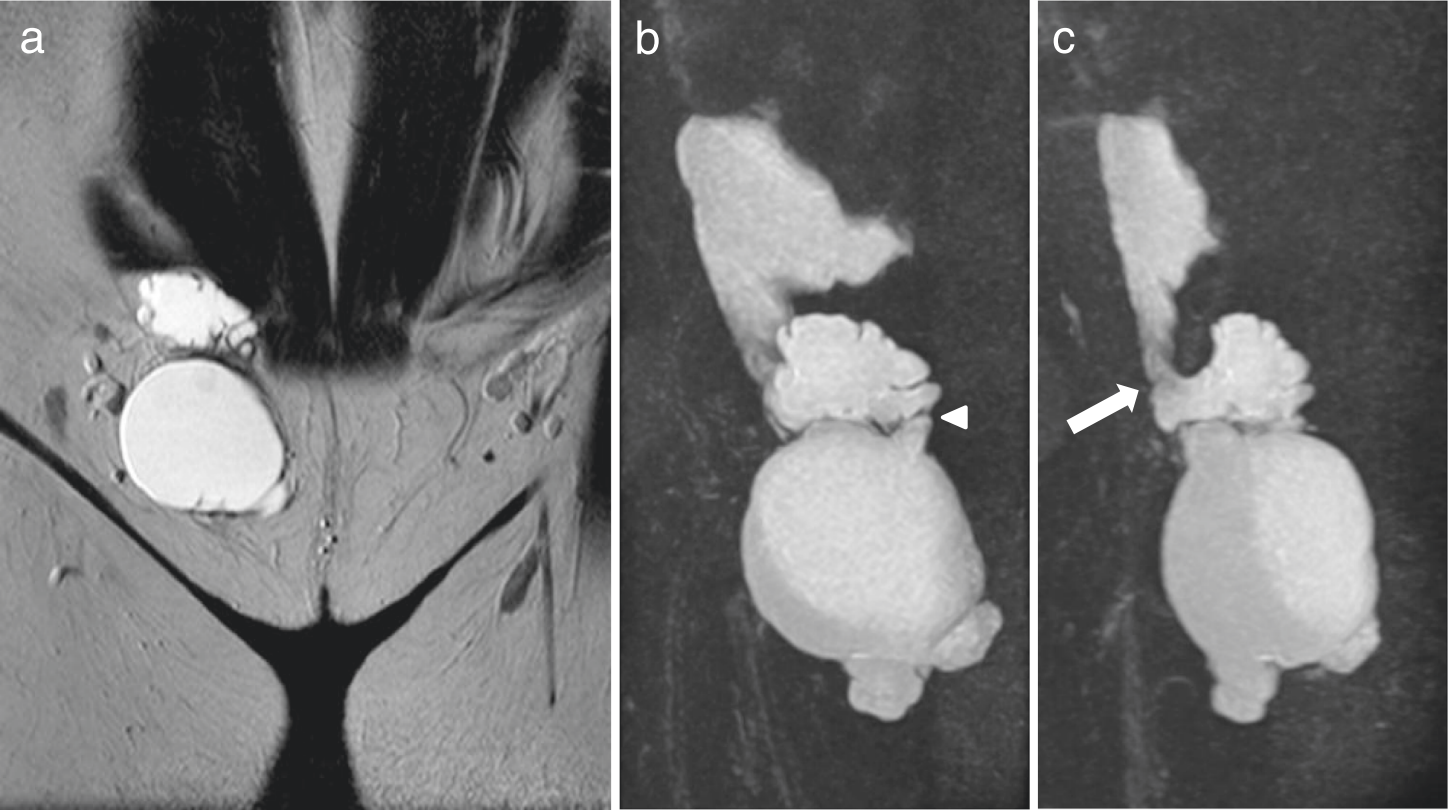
Coronal T2-weighted MRI and MR hydrography MIP images. Coronal T2-weighted MRI (a) and MIP images of MR hydrography [frontal view (b) and right anterior oblique view (c)] show an uninterrupted large cystic lesion extending from the pelvic cavity to the right inguinal region, measuring 13.5 cm in diameter with constrictions at the internal inguinal ring (*arrow*) and external inguinal ring (*arrowhead*)
